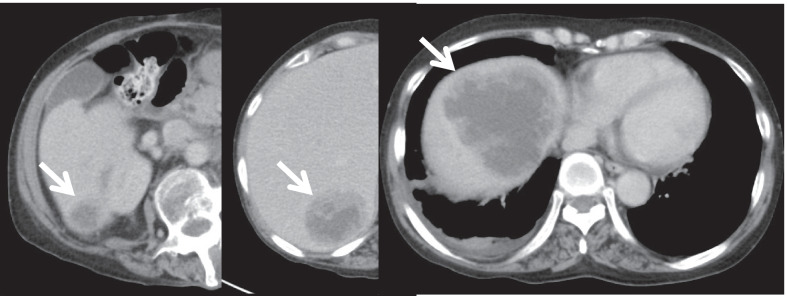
Abdominal CT scan at 3 months postoperatively showing marked increase in multiple liver metastases (white arrow). *CT* computed tomography
